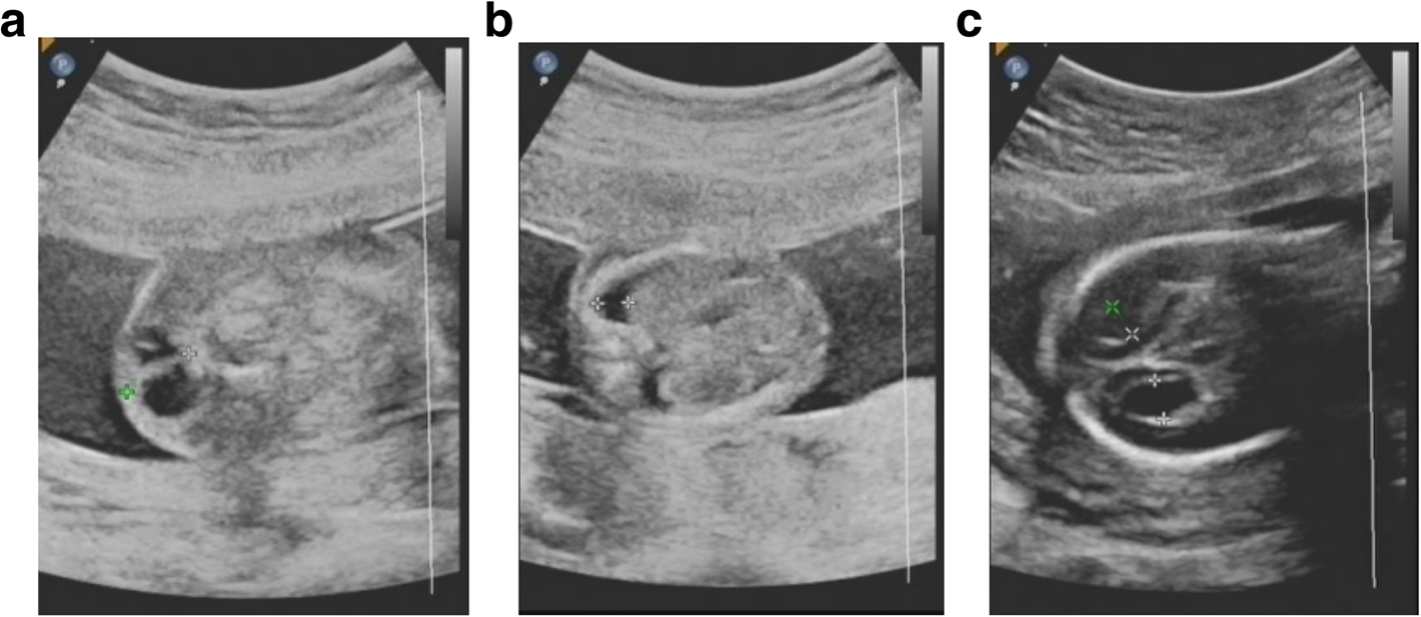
ultrasound findings of the first fetus. a increased nuchal fold with septation; b hydrothorax; c bilateral mild ventriculomegaly
